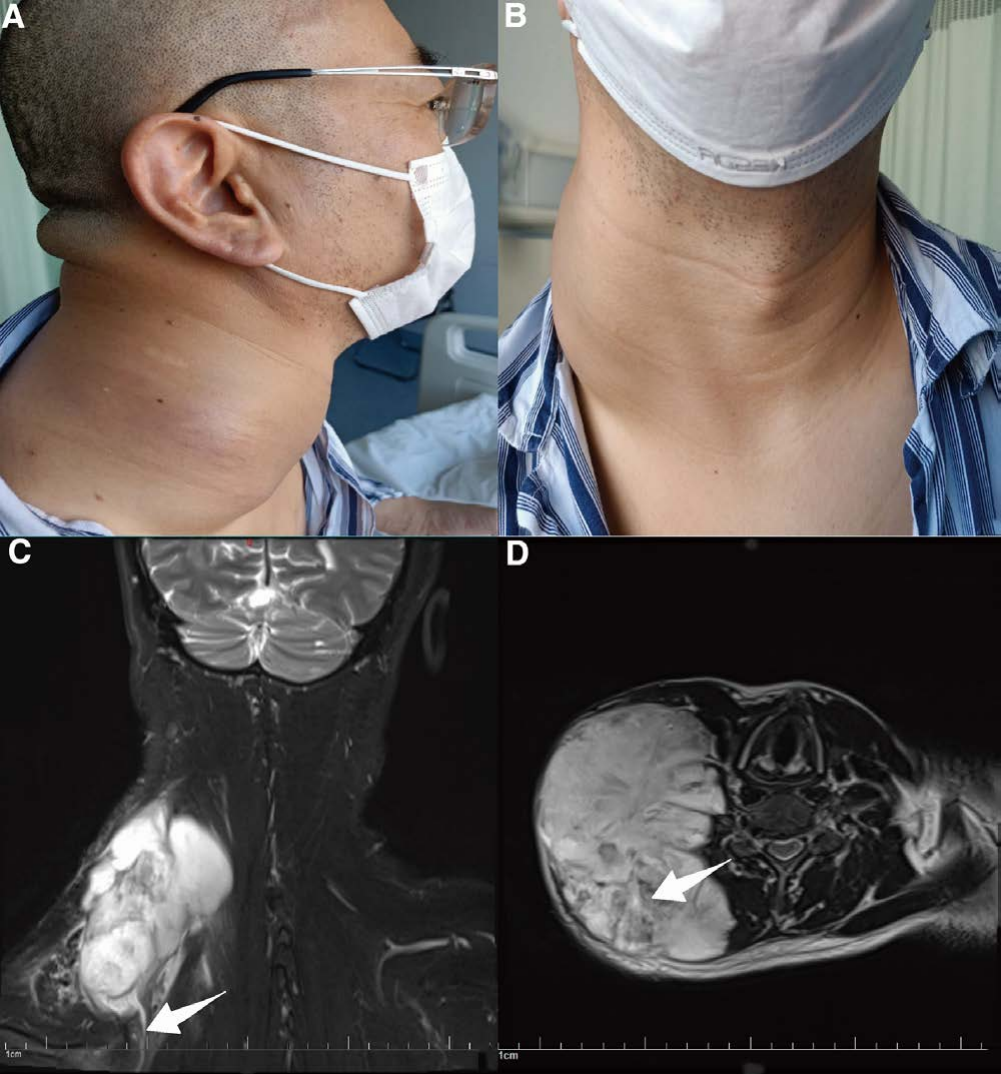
Images of clinical examination. (A, B) Clinical images showed a giant mass in the left neck. (C) MRI showed low signal intensity on T1WI and “tail sign” (arrow). (D) MRI showed high signal intensity on T2WI with separate pattern inside the tumor (arrow).
